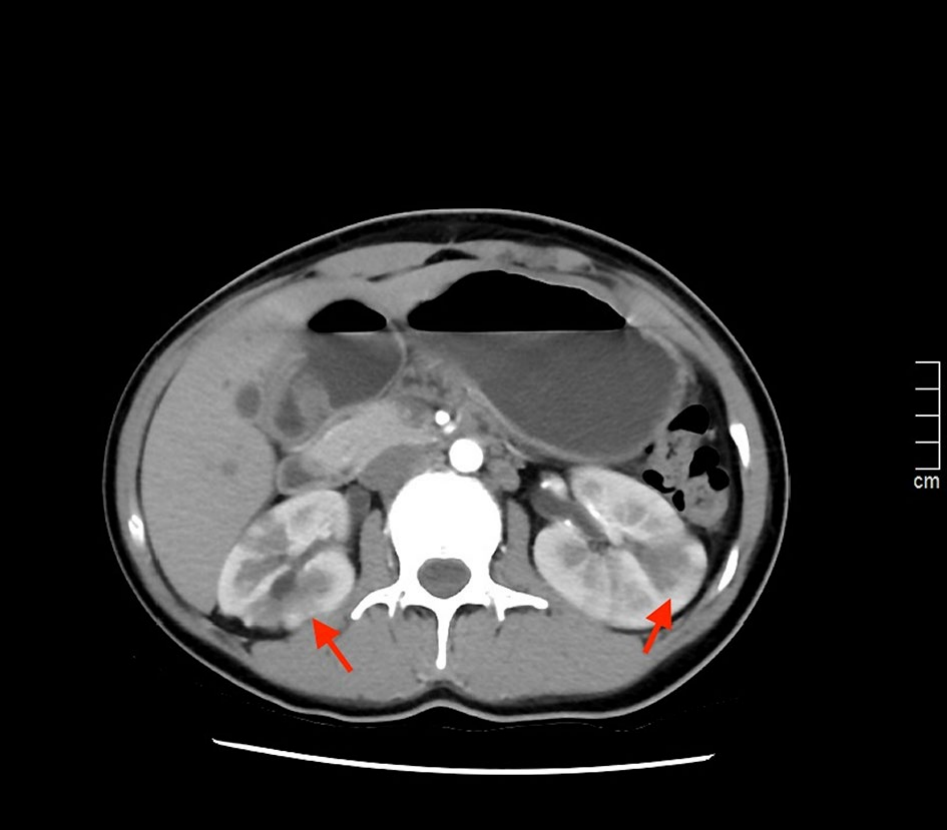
Typical findings for AFBN from contrast-enhanced CT. Images showing areas of wedge-shaped decreased enhancement in the left kidney and mass-like hypodense in right kidney (arrows).
